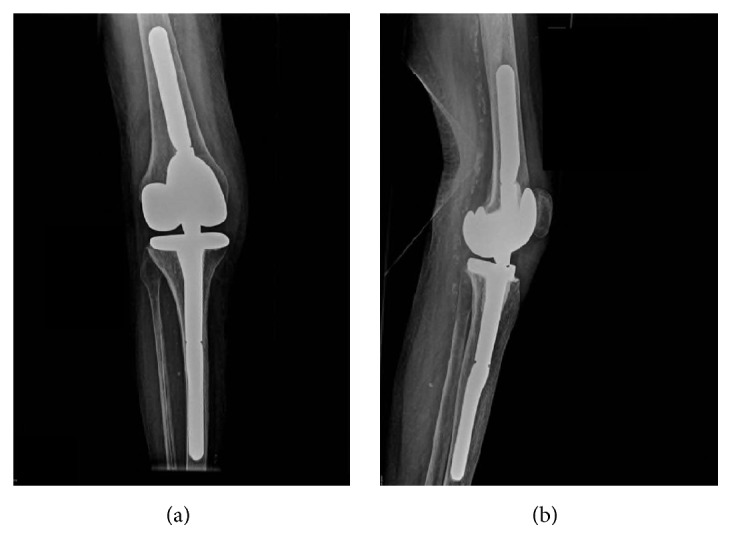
Postoperative standing X-rays of the knee: anteroposterior (a) and lateral (b) view. Tibiofemoral alignment of 6° valgus.
